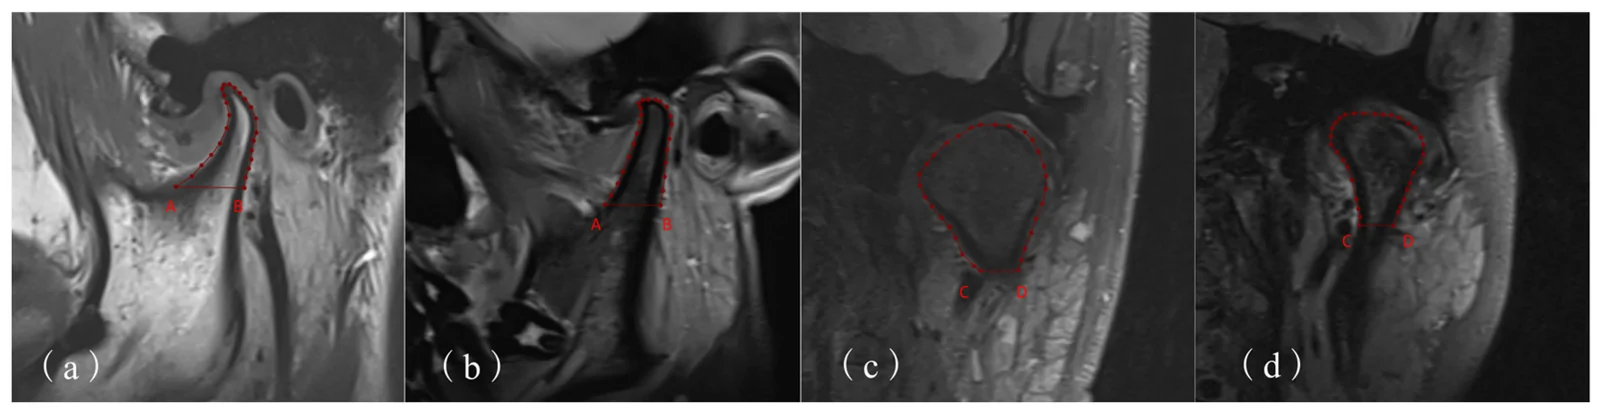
Annotation of the mandibular condyle on MRI images: (a,c) annotation method when the sigmoid notch is visible; (b,d) annotation method when the sigmoid notch is not visible.
